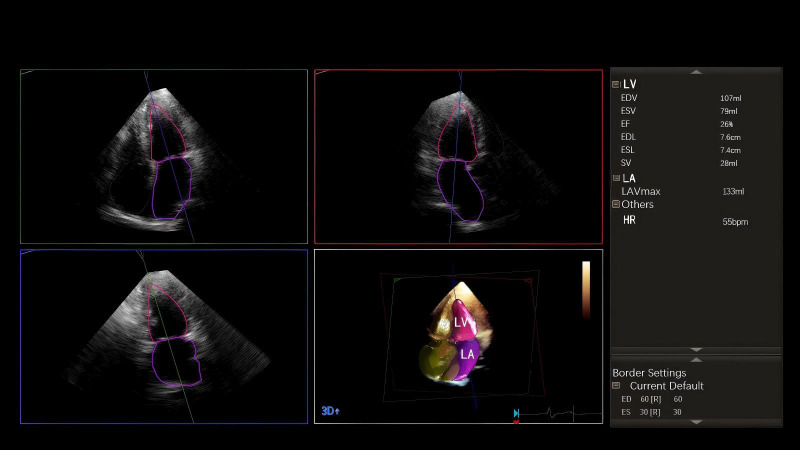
Apical four chamber view for a patient with HFrEF. Under the HM, the software automatically outlines the contours of the LA and LV and then analyzes and displays the following parameters: EDV (end diastolic volume), ESV (end systolic volume), 3D EF (ejection fraction), EDL (end diastolic diameter), ESL (end systolic diameter), SV (stroke volume), LAVmax (left atrial maximum systolic volume), and HR (heart rate).
